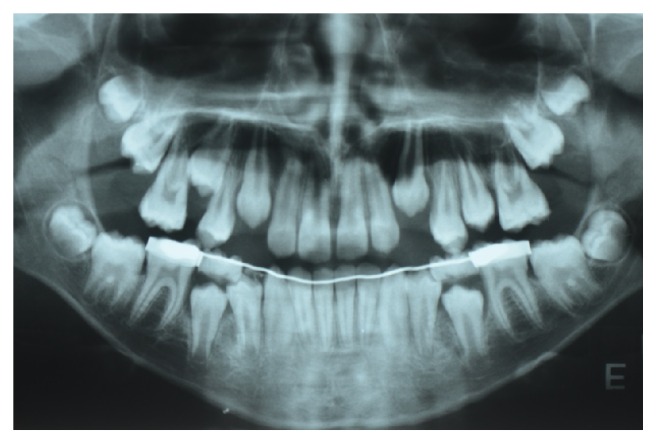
Panoramic radiograph of the patient aged 11 years and 10 months with no evidence of supernumerary teeth.
